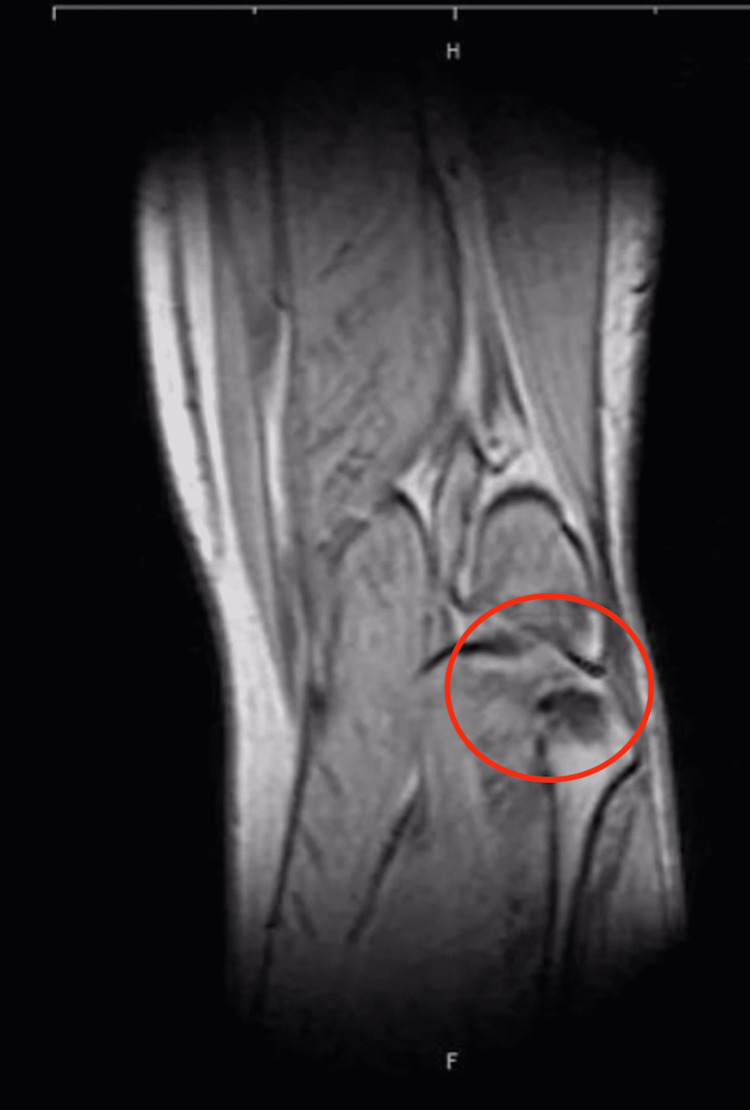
Preoperative MRI (T1 sequence) showing the osteoarthritis of the proximal tibiofibular joint
